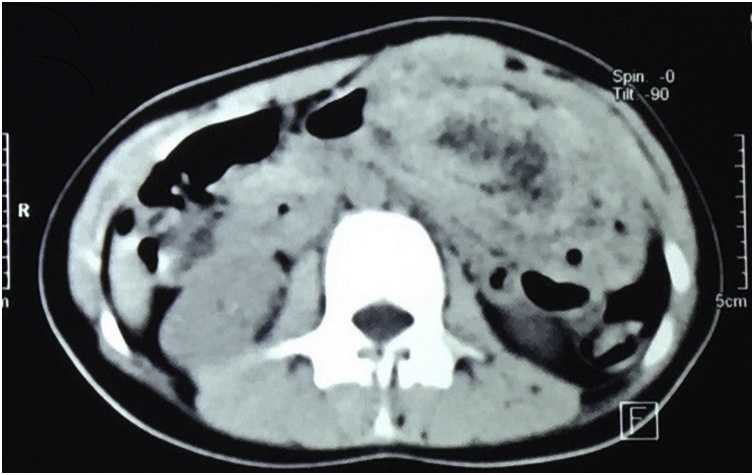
CT scan of the abdomen showing a large oval shaped intra-gastric mass with interspersed air.
